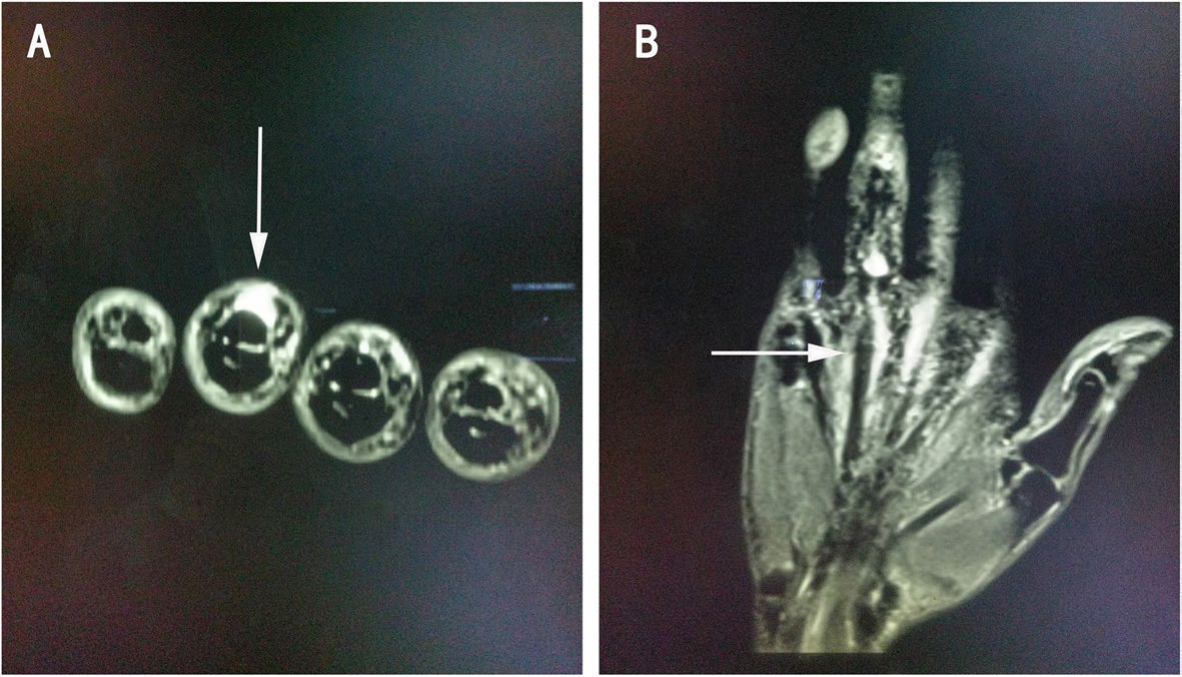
MRI T2-weighted image shows in-continuous high signal in finger extensor tendon (A) and edema in the surrounding tissue (B)
